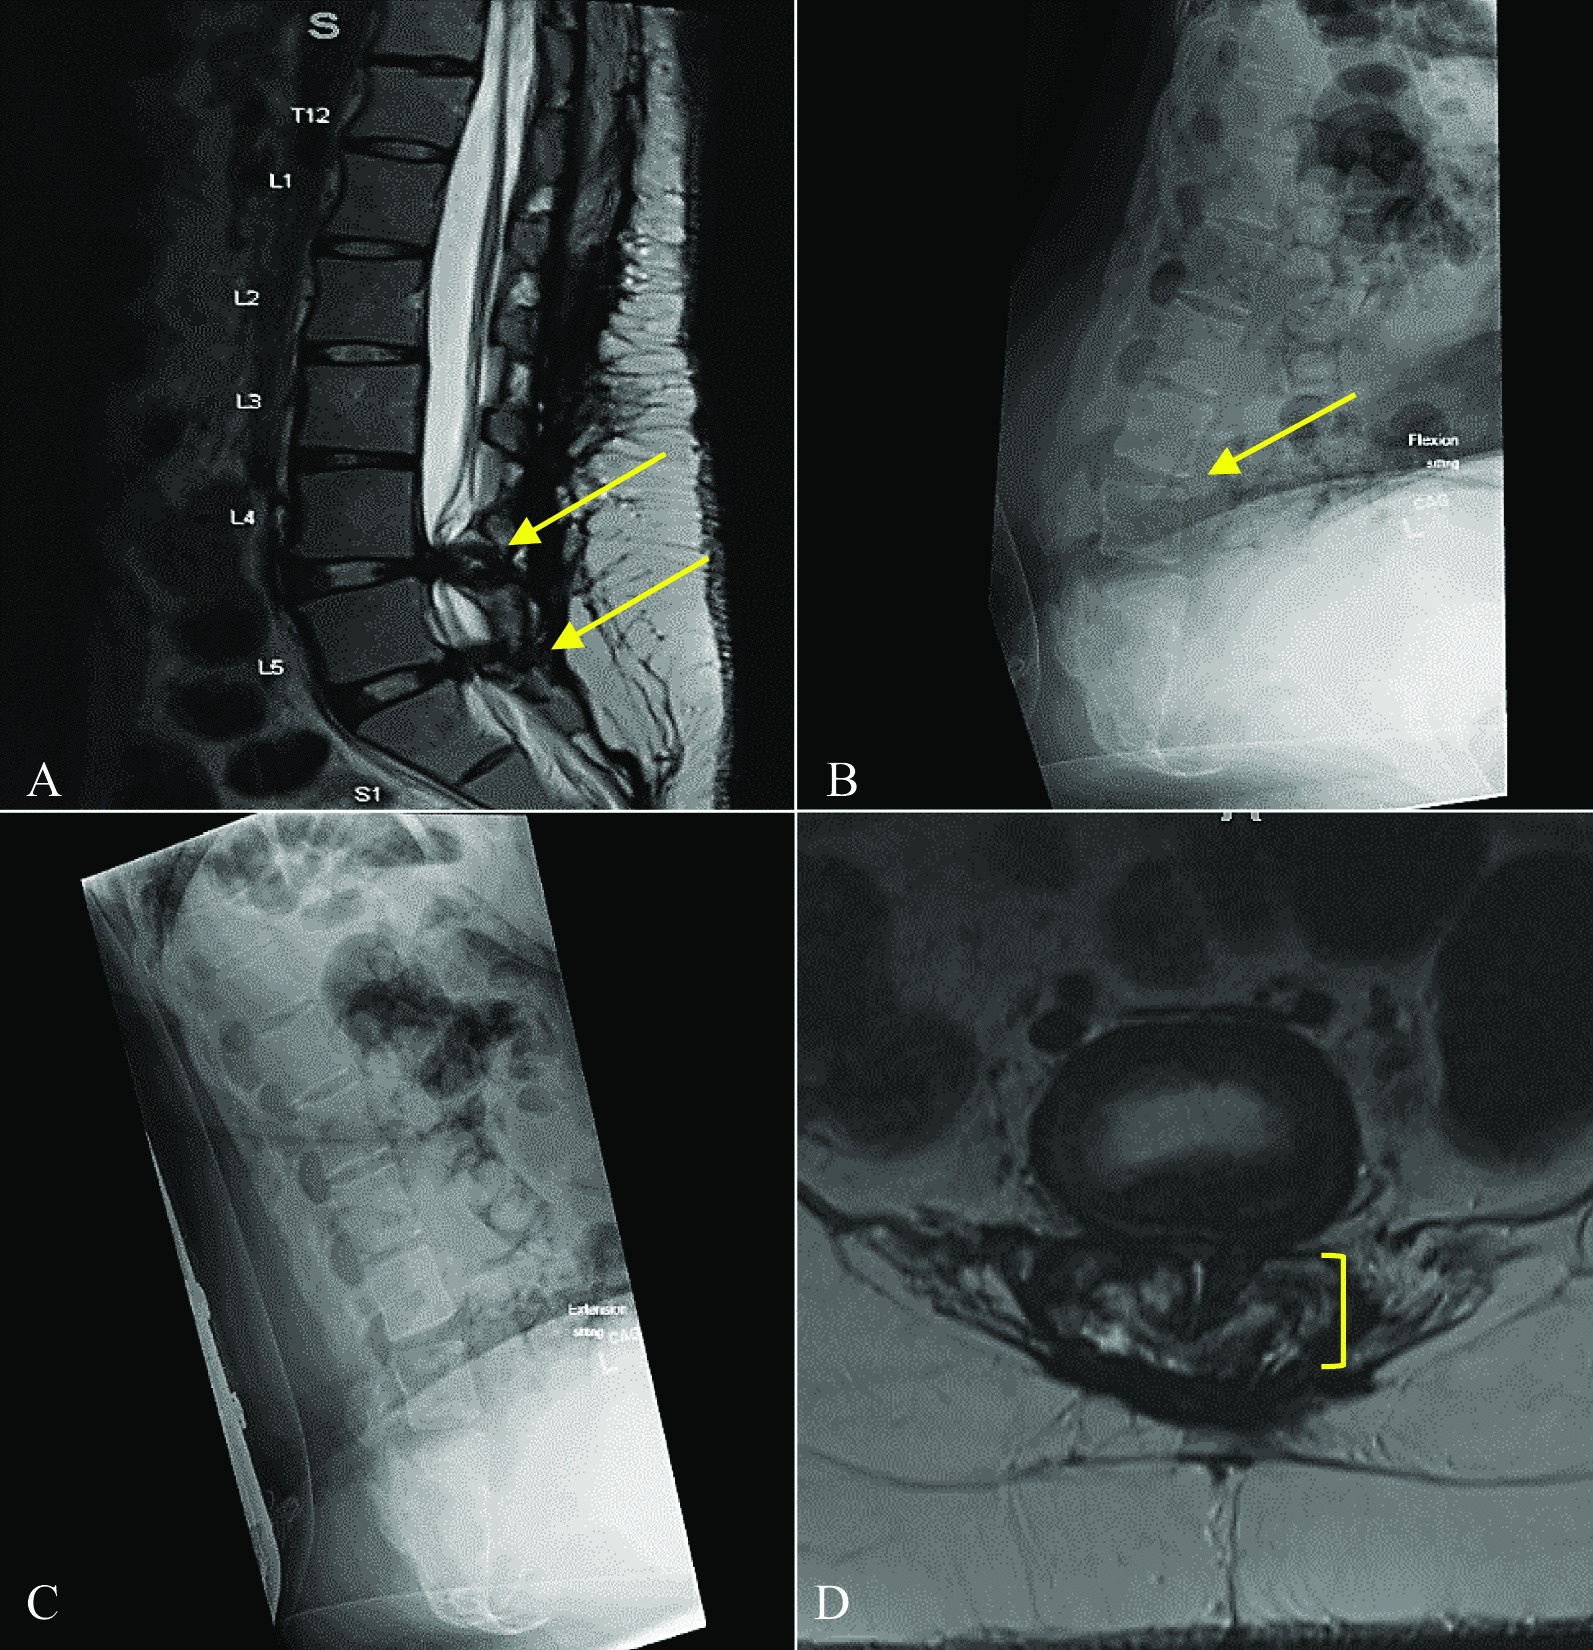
Preoperative images obtained prior to redo lumbar decompression and fusion surgery, ~ 80 months after diagnosis of TM and 30 months after removal of segmental instrumentation. a T2-weighted sagittal MRI of the lumbar spine demonstrating severe spinal stenosis at L4–L5 and L5–S1 (arrows) with near-total occlusion of the thecal sac. b Flexion lumbar radiograph showing loss of L4–L5 apposition without spondylolisthesis (arrow). c Extension lumbar radiograph showing improved alignment. d T2-weighted axial MRI of the L4–L5 disc space (bracket), demonstrating severe spinal stenosis
